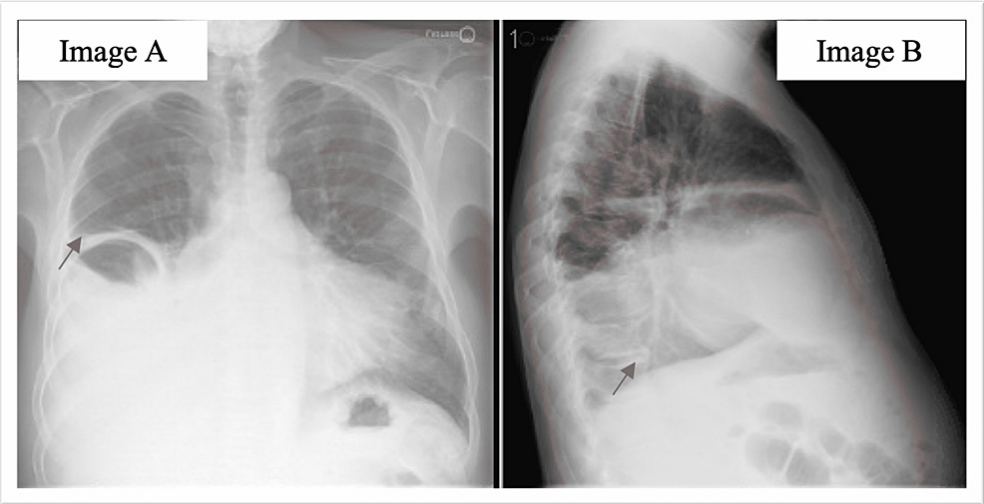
X-ray chest with red arrows in panels A and B showing large right pleural effusion with concerns for consolidation vs. atelectasis secondary to the effusion of the right lung base
